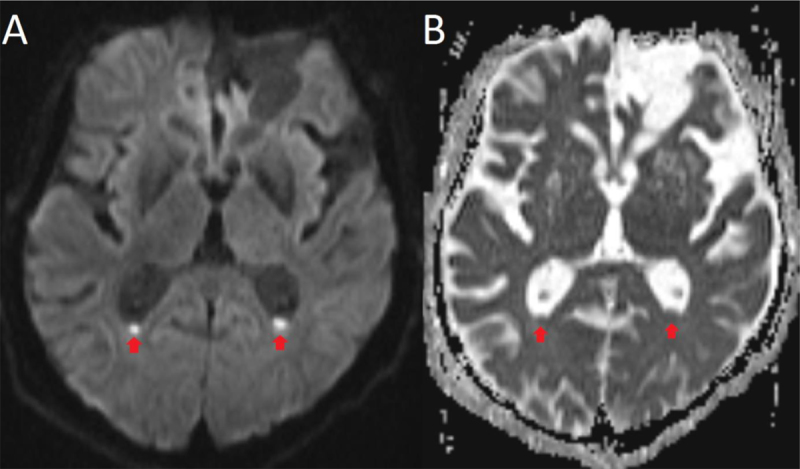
These ventricular sediments were slightly hypointense on T2-weighted images (T2WI, Figure 2A) and on fluid-attenuated inversion recovery (FLAIR, Figure 2B).
